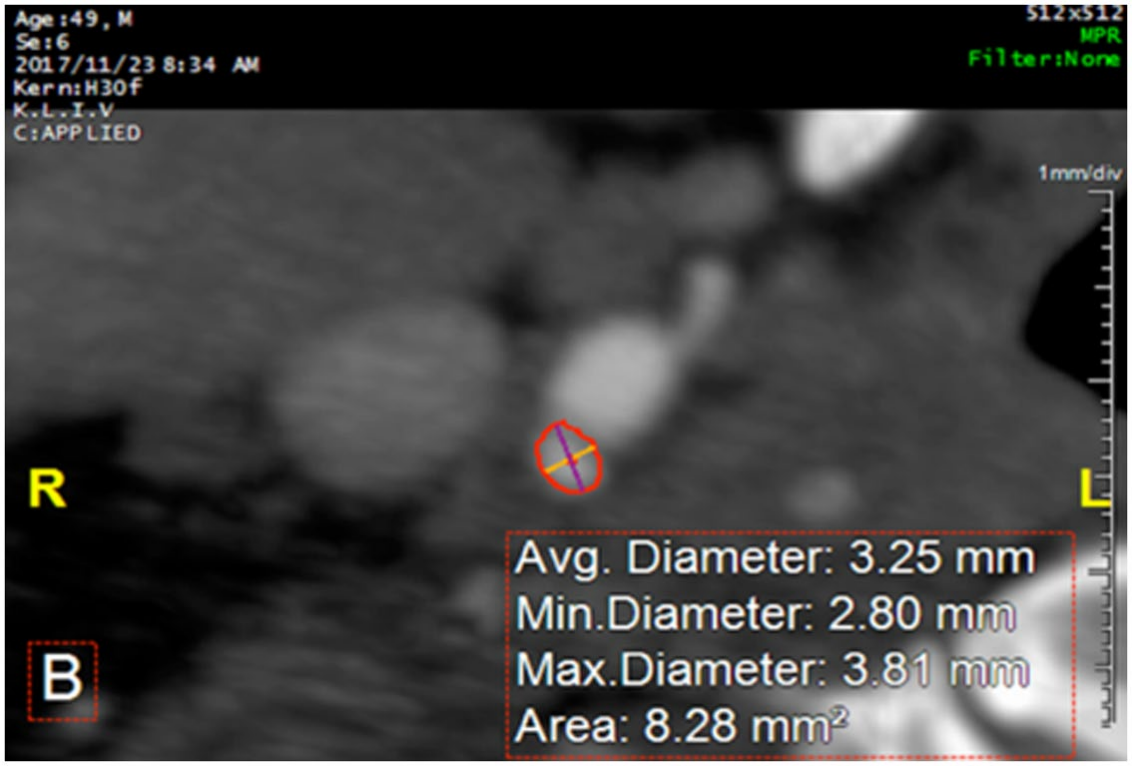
Measurement of the arterial lumen and percentage of stenosis using dedicated software on CTA.
